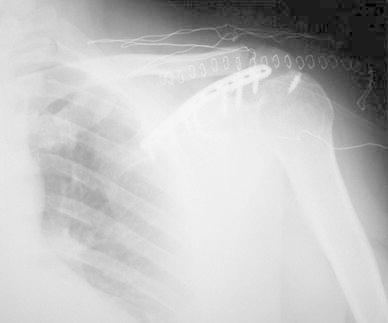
Early postoperative roentgenography
